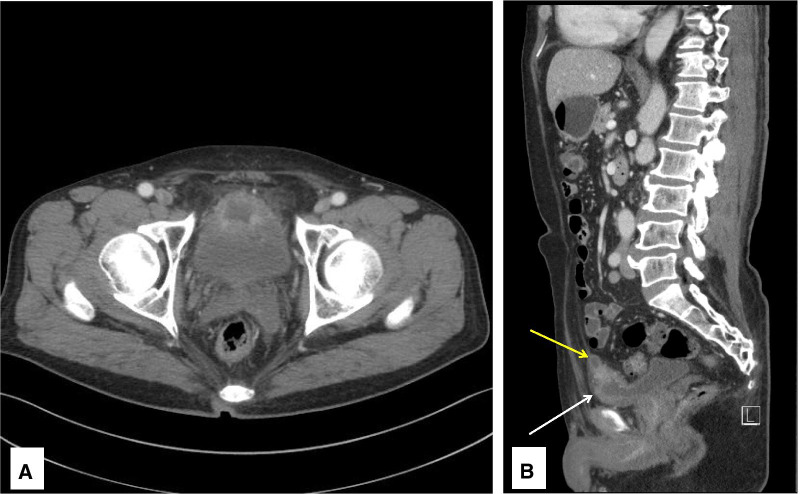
Follow-up computed tomography after transurethral resection shows heterogeneous enhancing mass, reduced in size, on the anterosuperior portion of the bladder. A Axial view of bladder mass. B Sagittal view of remnant bladder mass (white arrow) with an urachal cyst (yellow arrow)
